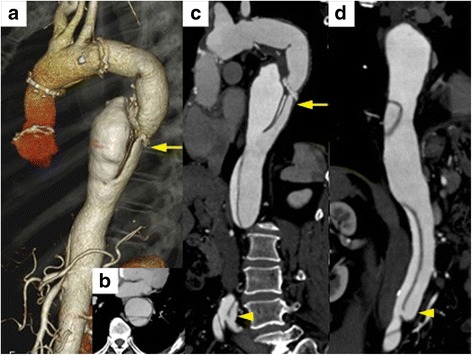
a Preoperative 3D–CTA imaging of a 59-year-old patient showing an aortic dissection with a 28-mm false lumen aneurysm and an 8-mm true lumen immediately above the celiac trunk. b An axial slice at the level of the arrow showing the aortic dissection with a 57-mm triple lumen aneurysm. c, d A saggital slice showing a major distal entry just above the aortic bifurcation (arrowhead)
